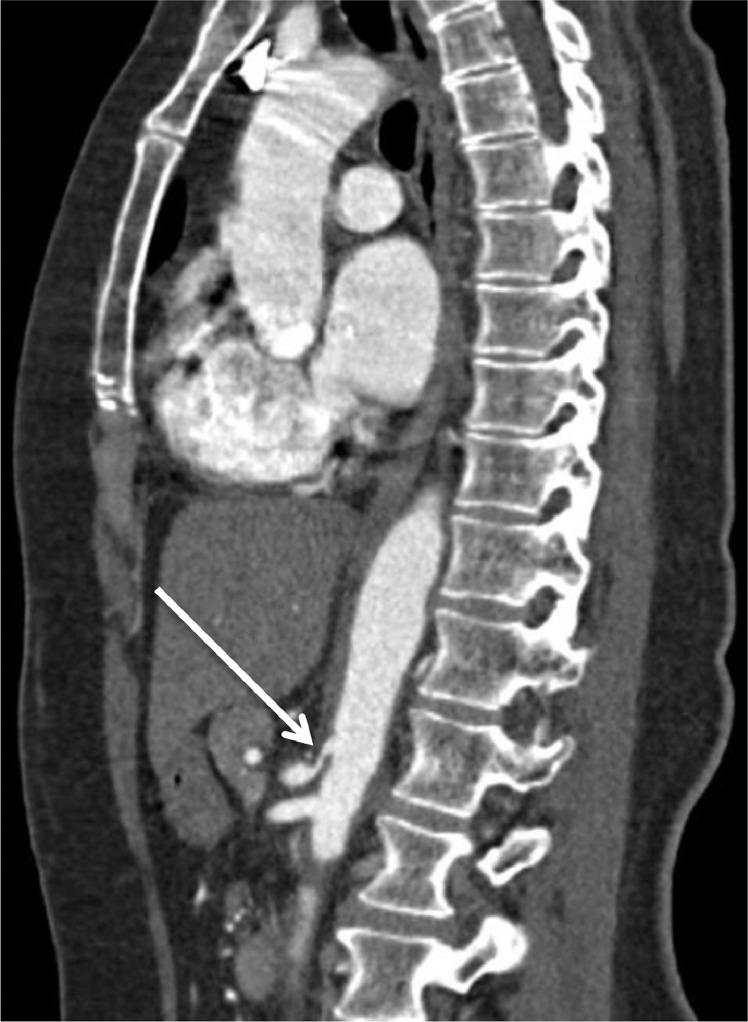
Sagittal CT of the aorta showing thickening of the wall of the aorta at the level of the celiac axis and superior mesenteric artery.
